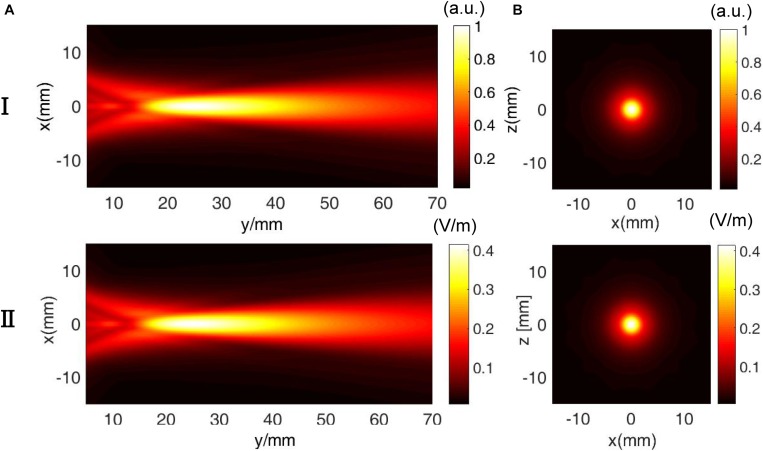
Comparison of the (I) ultrasound pressure field distribution and (II) electrical field intensity distribution at (A) the *x*–*y* section (*y* = 0 mm) and (B)
